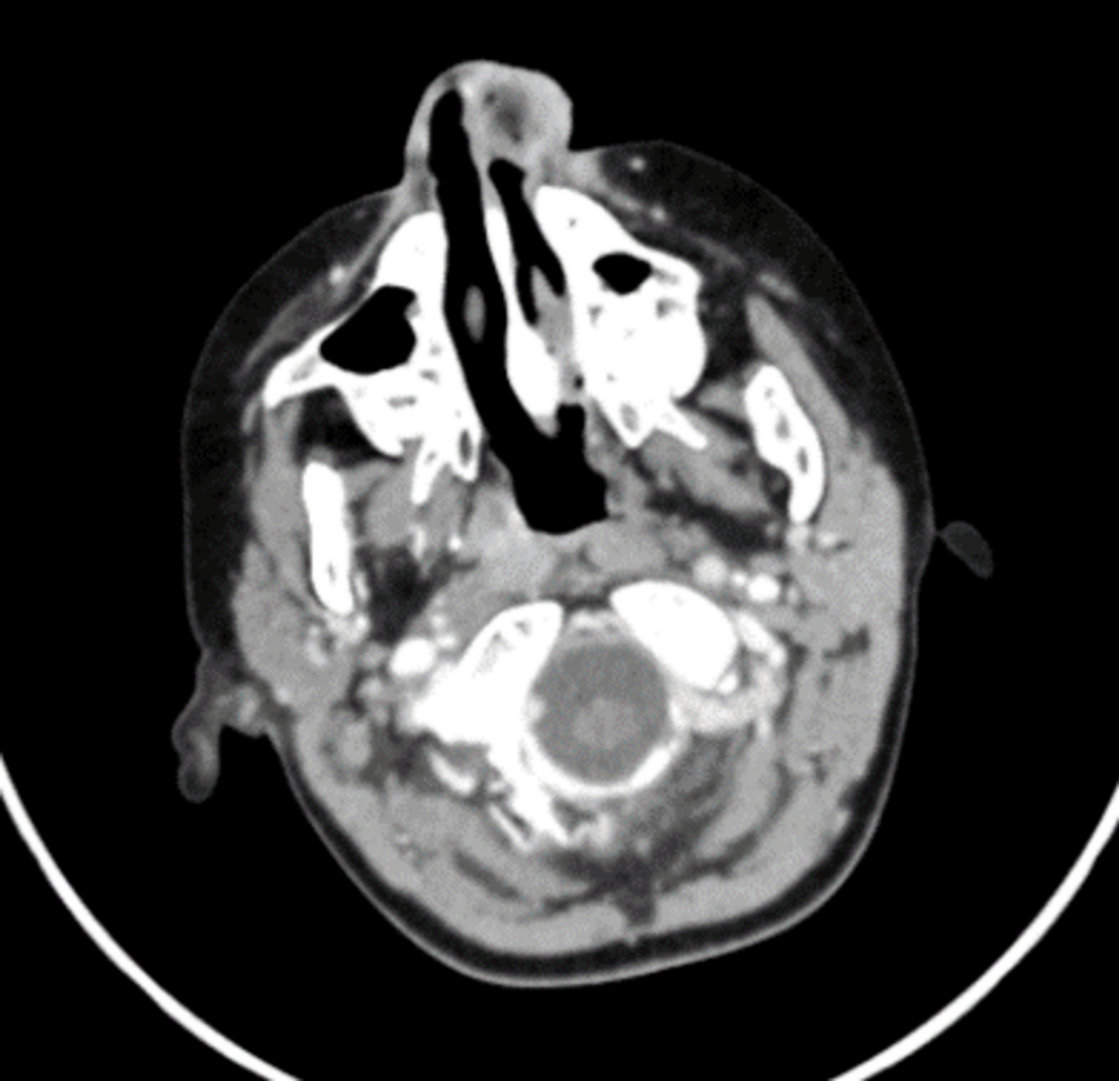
Contrast CT of the nasal herpetic lesion Subcutaneous thickening with inflammatory hyperenhancement at the left nasal alar and tip. CT: computed tomography
